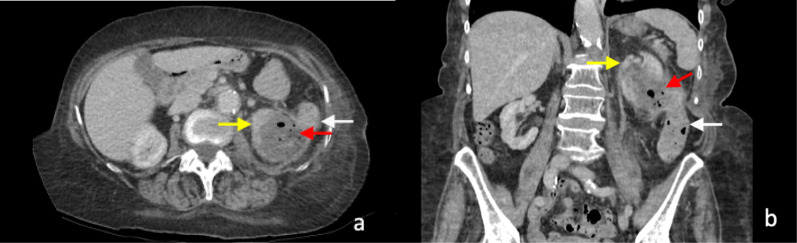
a Axial and b coronal enhanced CT images of a nephrocolonic fistula approximately 9 months following completion of 42 Gy of stereotactic ablative body radiotherapy of a left clear cell renal cell carcinoma. Faecal material is present within the fistulous connection (red arrow) between the descending colon (white arrow) and left kidney (yellow arrow)
